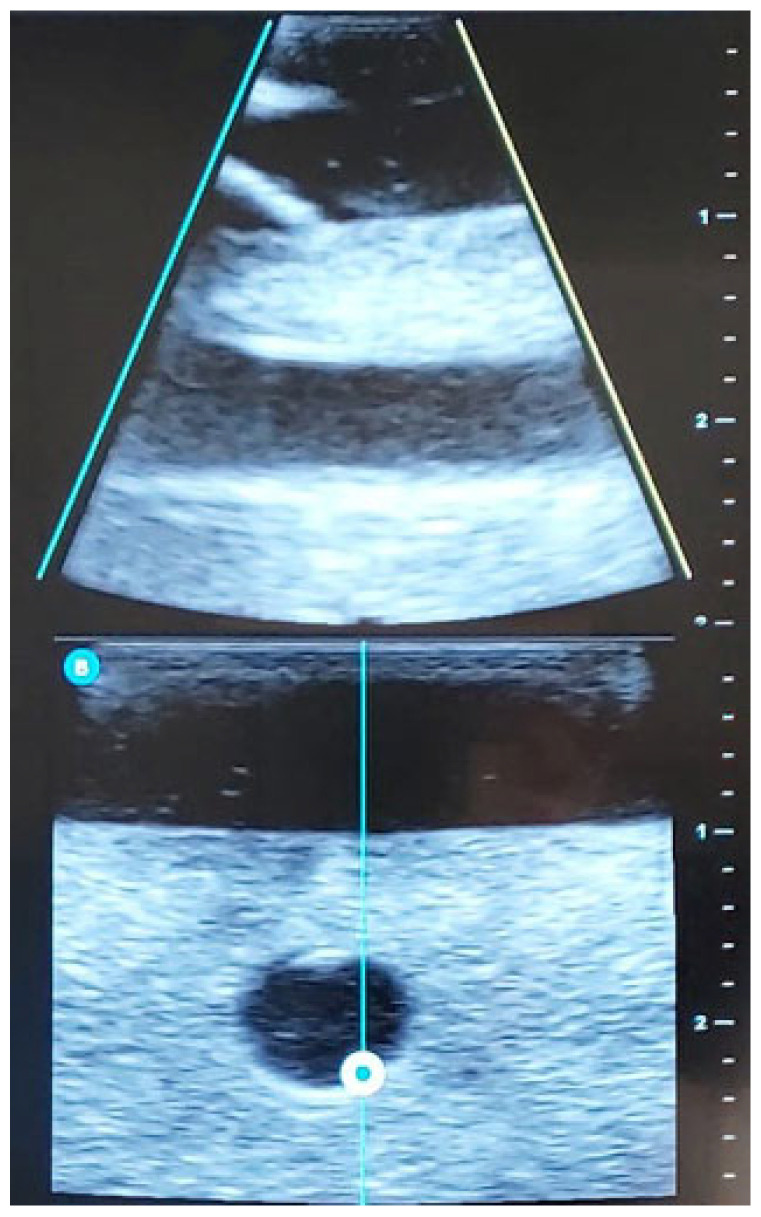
Ultrasound image of venous access. *Longitudinal plane. (Own source)*.
